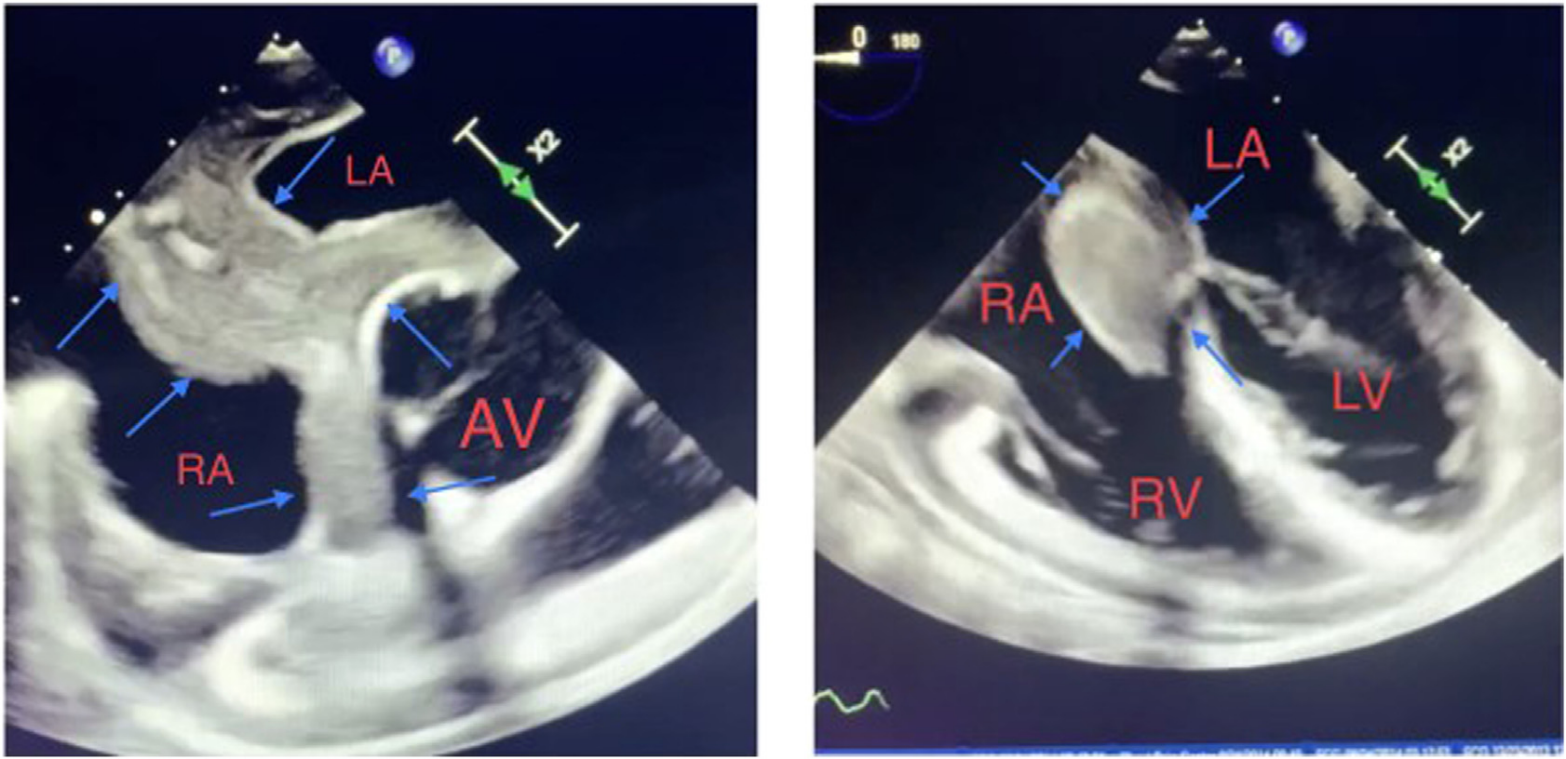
Transesophageal echocardiogram; blue arrows showing the abnormal mass involving the interatrial septum. AV = aortic valve; LA = left atrium; LV = left ventricle; RA = right atrium; RV = right ventricle.
